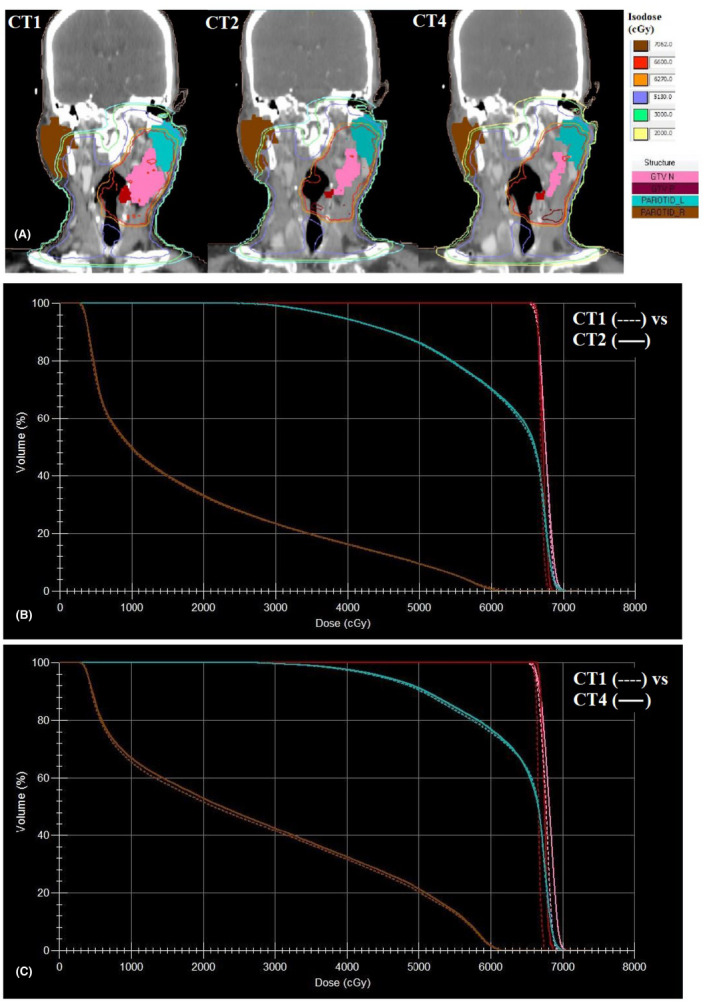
A, Coronal CT sections of a 55‐years‐old carcinoma pyriform fossa (T1N3b) patient. The gradual decrease in GTV primary (maroon), GTV nodal (pink) and bilateral parotid gland volumes can be appreciated. The low dose isodose curves [20 Gy (light yellow) and 30 Gy (light green)] can be seen covering more areas of right parotid gland in CT4 compared to CT1 and CT2. b. Comparative DVH of CT1 (‐‐‐‐‐‐‐) and CT2 (
